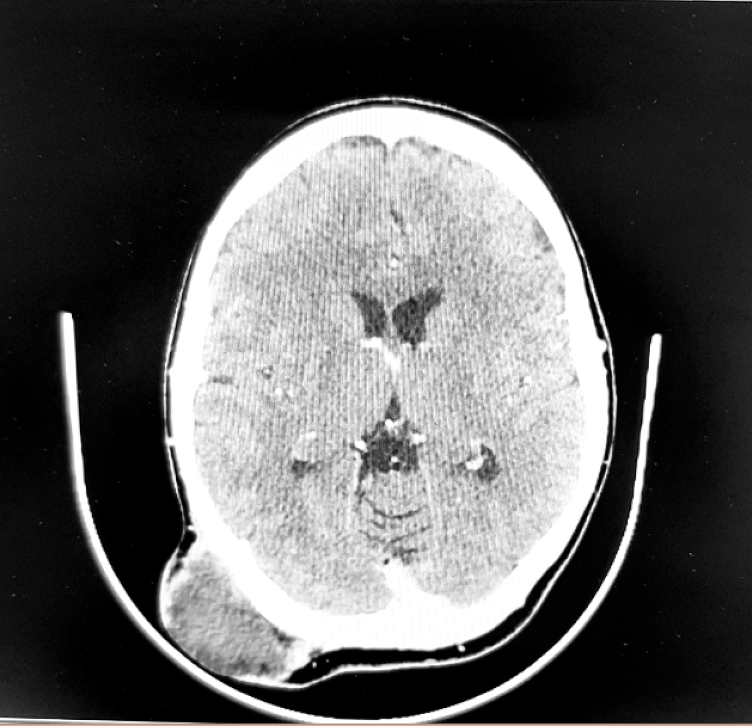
Brain CT scan of patient with Ewing's sarcoma.
